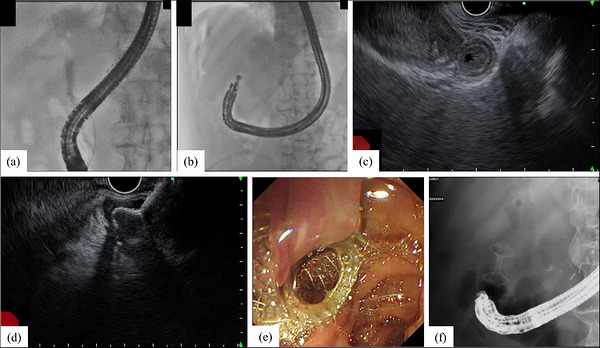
Rescue endoscopic ultrasound (EUS)‐guided gallbladder drainage in a patient with Billroth‐I reconstruction after failed endoscopic transpapillary gallbladder drainage. (a) Fluoroscopic image showing attempted cystic duct cannulation during endoscopic retrograde cholangiopancreatography; the cystic duct branched downward and was suspected to have been narrowed due to inflammation, preventing guidewire advancement into the gallbladder. (b) Fluoroscopic image demonstrating echoendoscope positioning in a patient with Billroth‐I reconstruction. (c) Endoscopic ultrasound image showing a distended gallbladder with stones. (d) Deployment of the distal flange of the 10‐mm lumen‐apposing metal stent within the gallbladder under EUS guidance. (e) Endoscopic view of the deployed lumen‐apposing metal stent with drainage of infected bile. (f) Fluoroscopic image confirming appropriate stent positioning.
